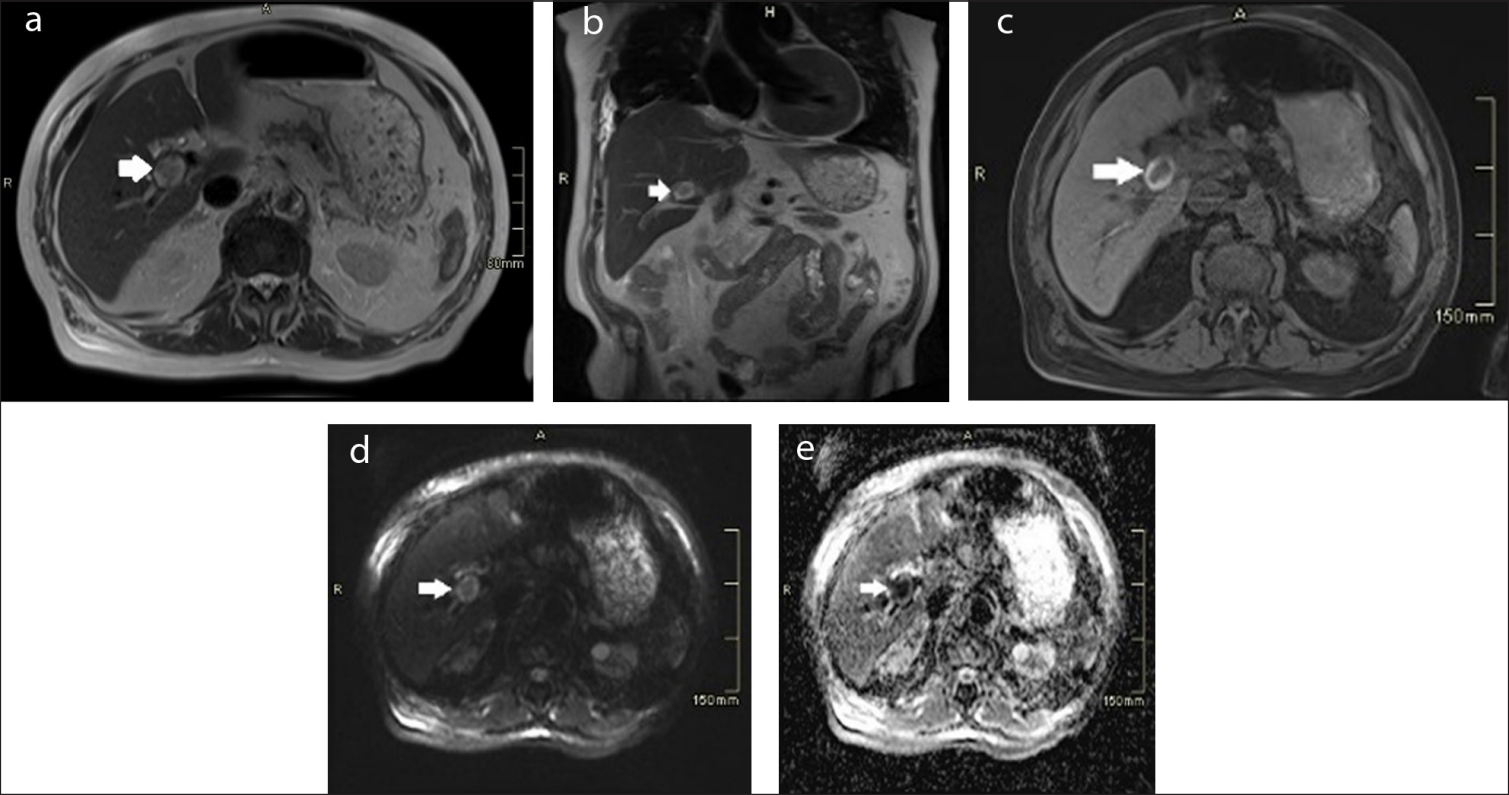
Axial (a) and coronal (b) fat-sat T2 and axial T1 (c) sequence show a lesion with a hyperintense periphery and a hypo-intense centre (white arrows). High signal intensity on the DWI (d) and low signal on ADC (e) is noted, confirming diffusion restrictive lesion (white arrows).
